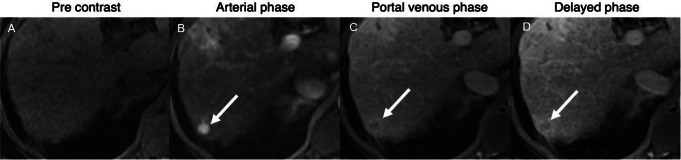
HCC on DCE-AMRI. Axial pre-contrast (A) and contrast-enhanced arterial phase (B), portal venous phase (C), and delayed phase (D) T1-weighted MR images show a small isointense lesion in the right hepatic lobe which demonstrates arterial phase hyperenhancement, washout, and capsule diagnostic of HCC
